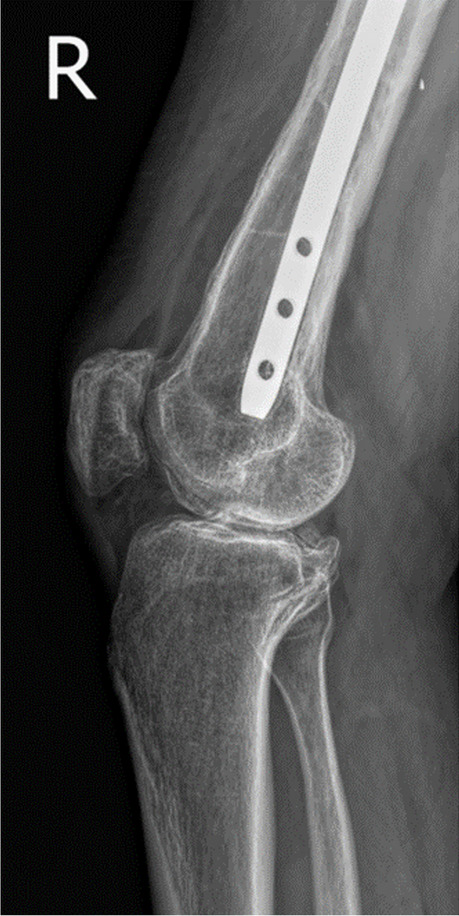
X-ray taken at 31 months follow-up
